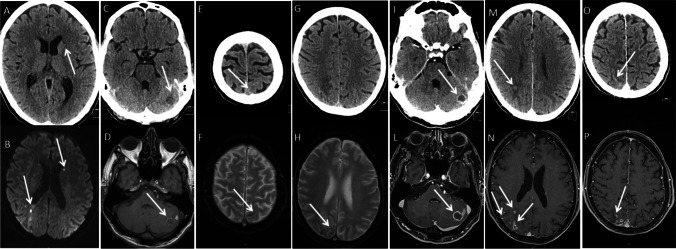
This figure summarizes the most common types of lesions identified in our study in a representative case who underwent both CT (top row) and MRI (bottom row), both plain (panels A-H) and enhanced (panels I-P). The following MRI sequences are shown: Diffusion-weighted image (DWI) (panel B), T2* weighted Gradient echo (GRE) (panels F, H), pre-contrast T1 weighted GRE (panel D) and post-contrast T1 weighted GRE (panels L-P). Arrows indicate punctuate ischemias (panel A, B), hemorrhages (panels C, D), subarachnoid hemorrhages (panels E, F), cerebral microbleeds (panel H, not clearly visible in G), abscesses (panels I, L), microabscesses (panels M, N), meningitis (panels O, P)
